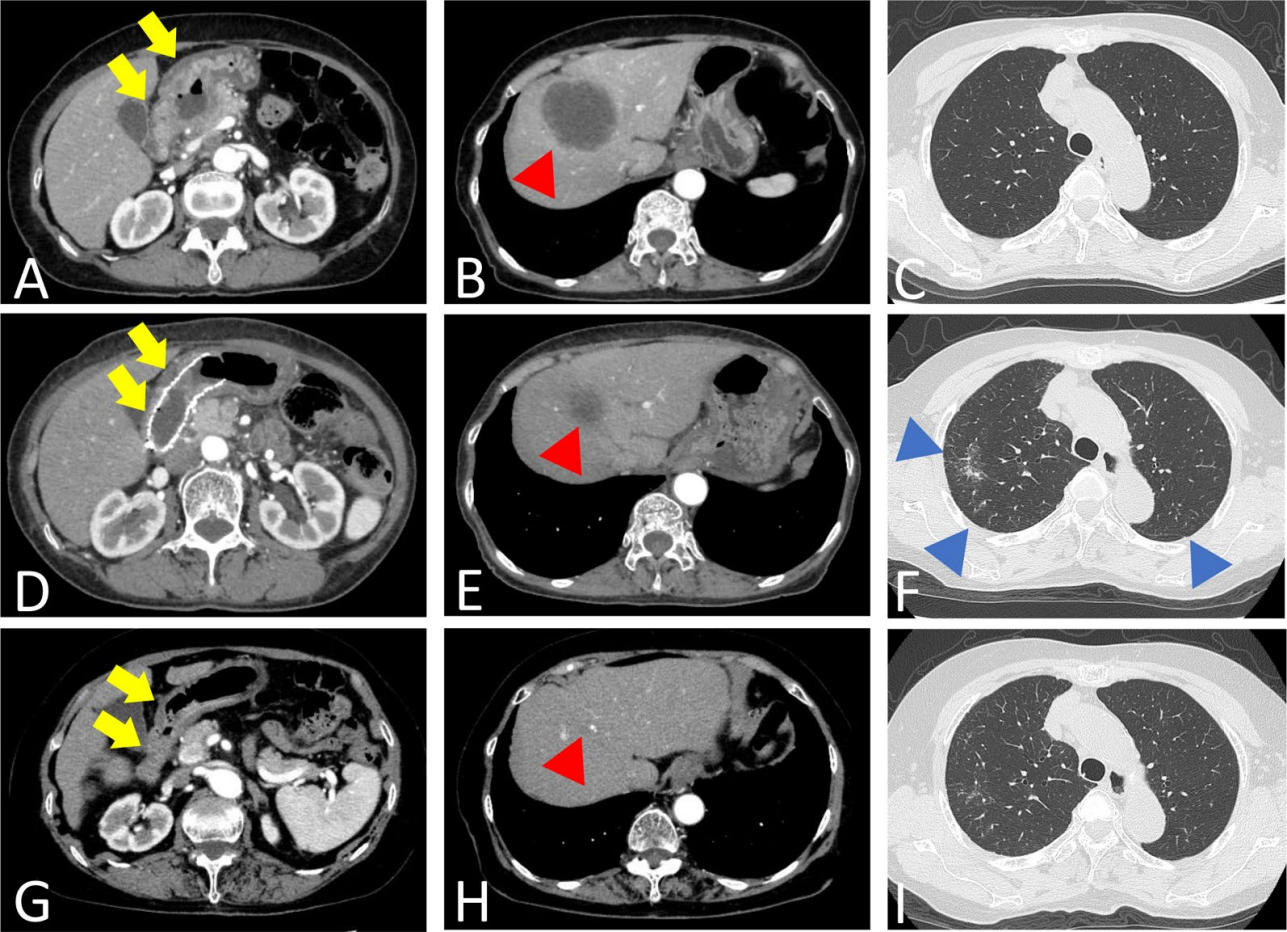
Computed tomography (CT) in Case 1. Arrows, primary gastric tumor; red arrowheads, liver metastases; blue arrowheads, lesions of interstitial pneumonia. A Before starting chemotherapy, the gastric tumor extends from the lower body to the pylorus of the stomach. B Liver metastases of up to 6.0 cm are observed. C CT of the lungs reveals no obvious lesions. D CT shows a reduction in the primary tumor after 3 cycles of SOX therapy. However, after two cycles, a metallic stent is inserted into the tumor. E CT shows a reduction in the liver metastases after 3 cycles of SOX therapy. F CT after 44 cycles of SOX therapy shows reticular and ground-glass opacities in both lung fields. G Sixty-six months after starting chemotherapy, CT shows significant shrinkage of the primary tumor. The metallic stent migrated and disappeared from the gastric pylorus. H CT shows significant shrinkage of the liver metastases. I Three months after stopping SOX therapy, CT shows an improvement of the interstitial lesions in both lungs
